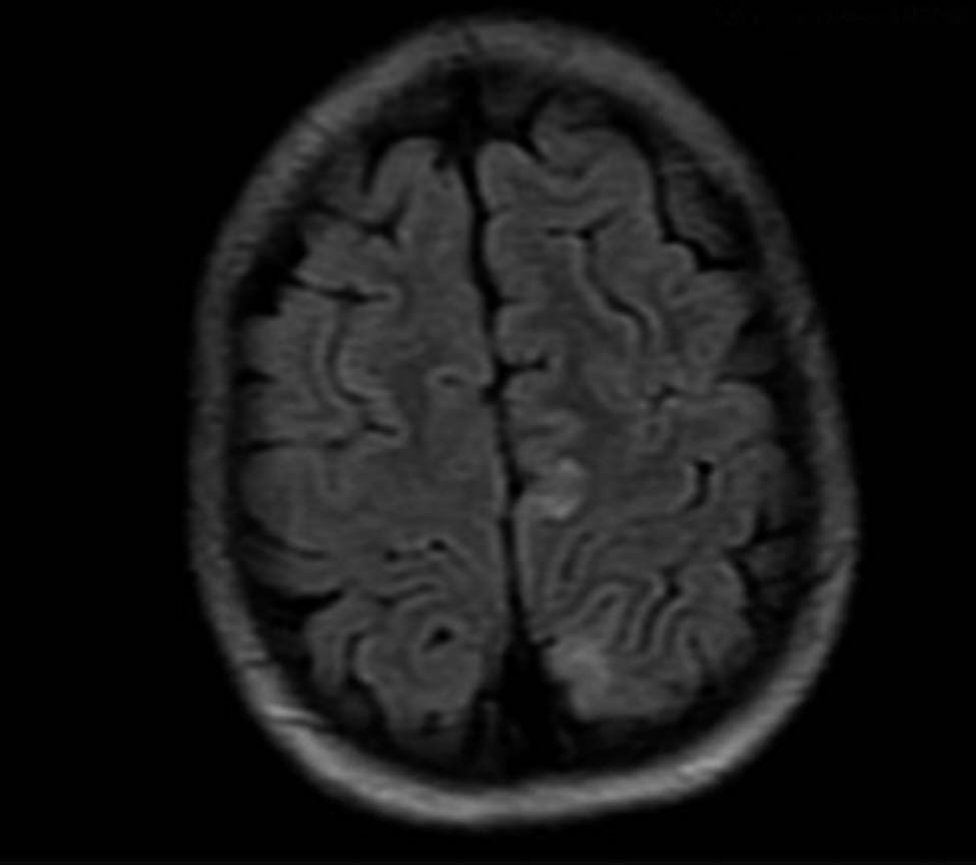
Axial view of MRI Brain showing subtle FLAIR hyperintensities in left frontal and bilateral parietal cortical sulci and subcortical white matter.
